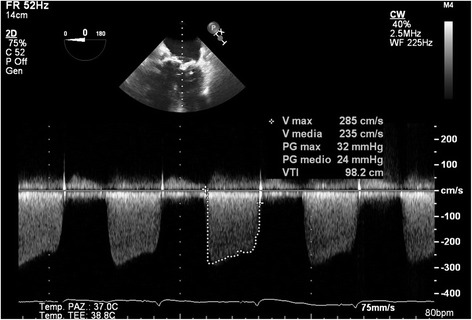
Preoperative transthoracic echocardiography showing severe mitral stenosis (transvalvular mean gradient 24 mmHg)
